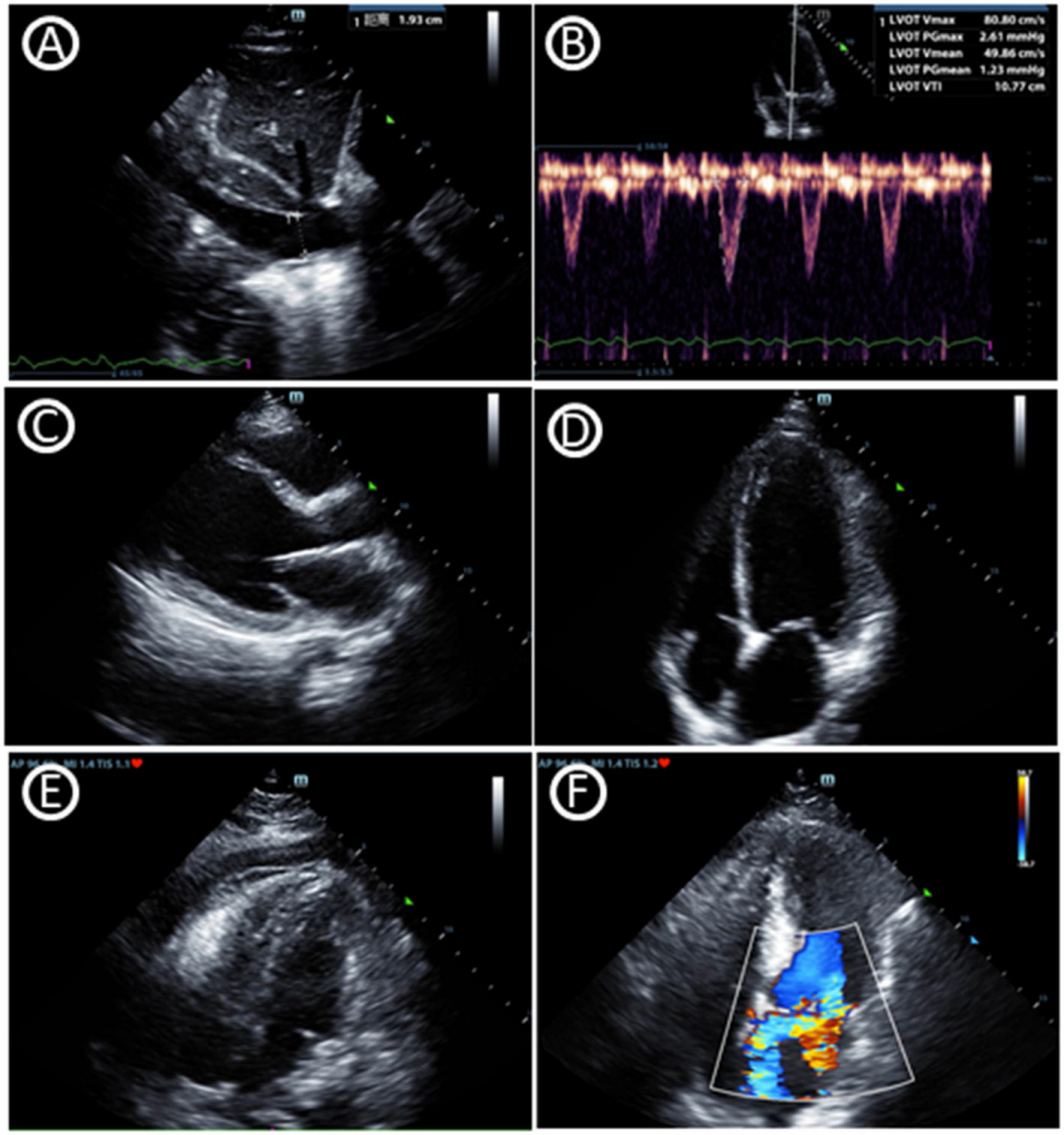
Critical care echocardiography imaging. (A) Inferior vena cava diameter. (B) Velocity-time integral of left ventricular outflow tract, which can help to gain cardiac output and fluid responsiveness. (C) Parasternal window parasternal long-axis view. (D) Apical window four-chamber view. (E) Pericardial effusion in subcostal window four-chamber view. (F) Mitral regurgitation in apical window four-chamber view.
